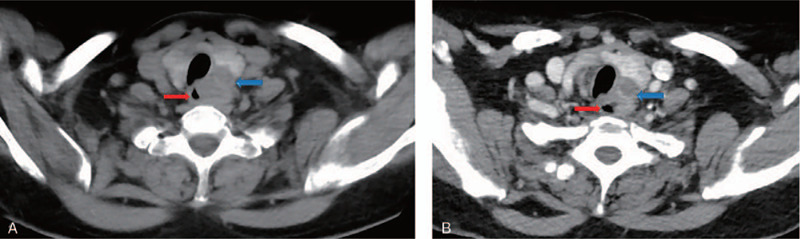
CT imaging demonstrating the improvement of esophageal compression and tumor reduction. (A) Scan after 1 month of apatinib. (B) Scan after 6 weeks of apatinib. CT = computed tomography.
